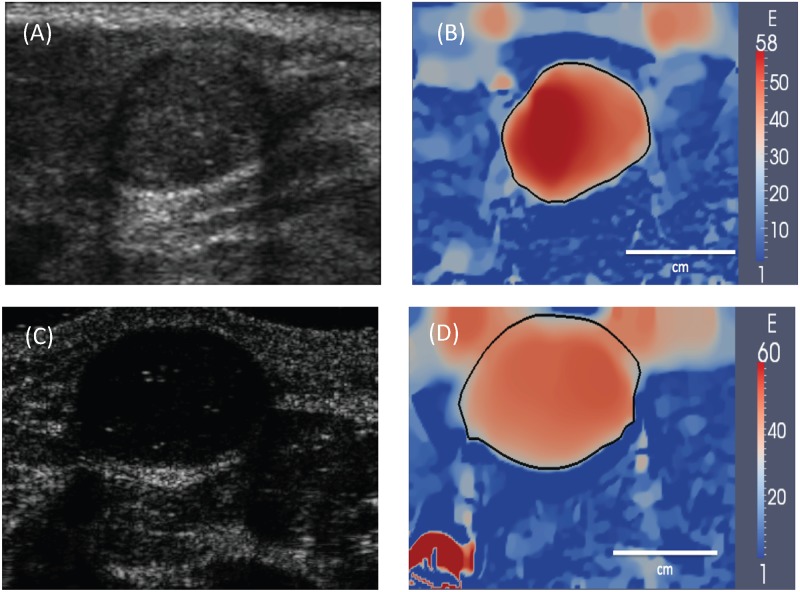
(A) and (C): B-mode ultrasound images of two typical fibroadenomas. (B) and (D): Corresponding Young’s modulus images generated using elasticity imaging. The tumor boundary is represented by a black curve that is drawn using 50 modulus value. The modulus distribution within the tumors is relatively homogeneous and the margins of the tumor are smooth.
